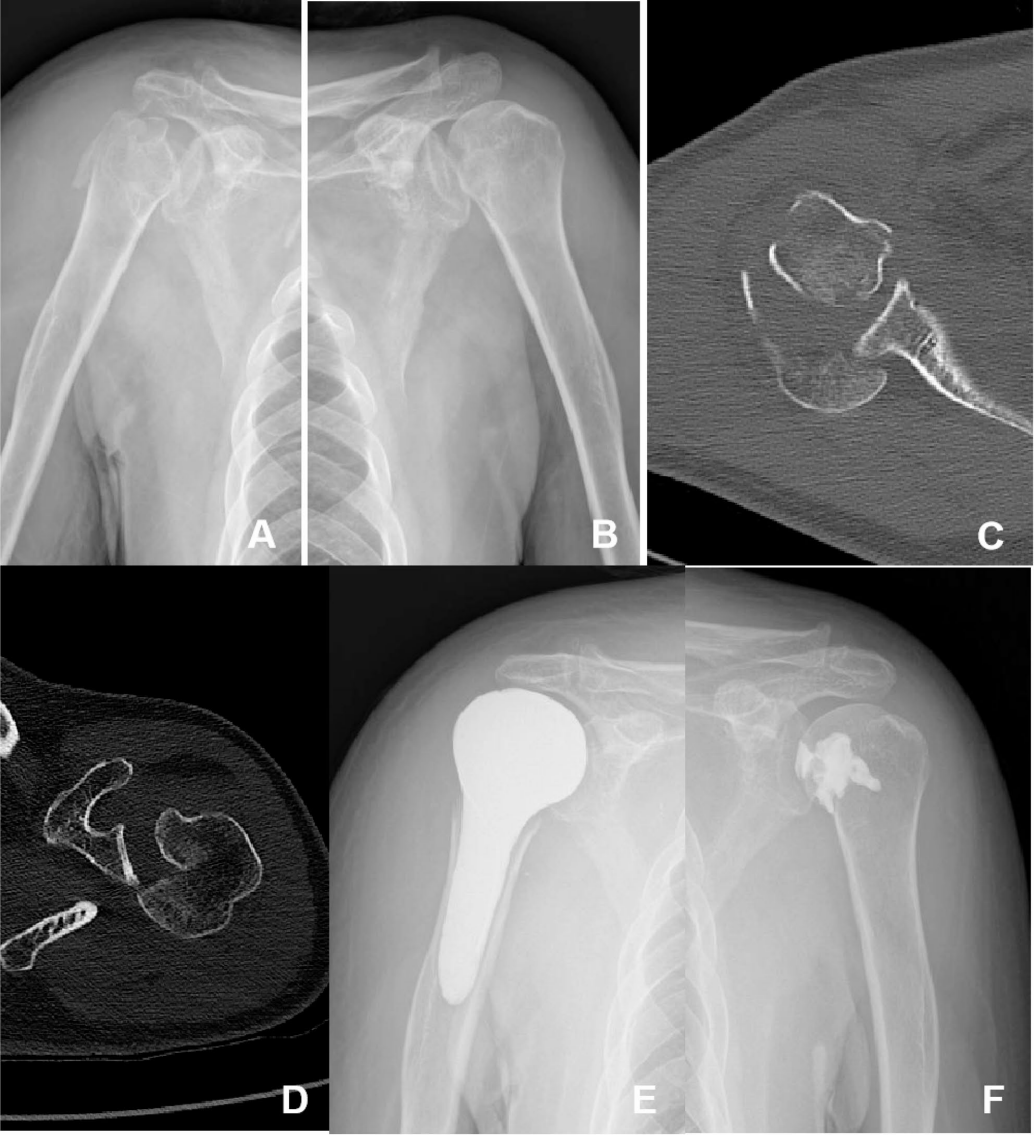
Images of Case 2. A AP view of the right shoulder; B AP view of the left shoulder; C CT scan of the right shoulder; D CT scan of the left shoulder; E Postoperative AP view of the right shoulder; F Postoperative AP view of the left shoulder
